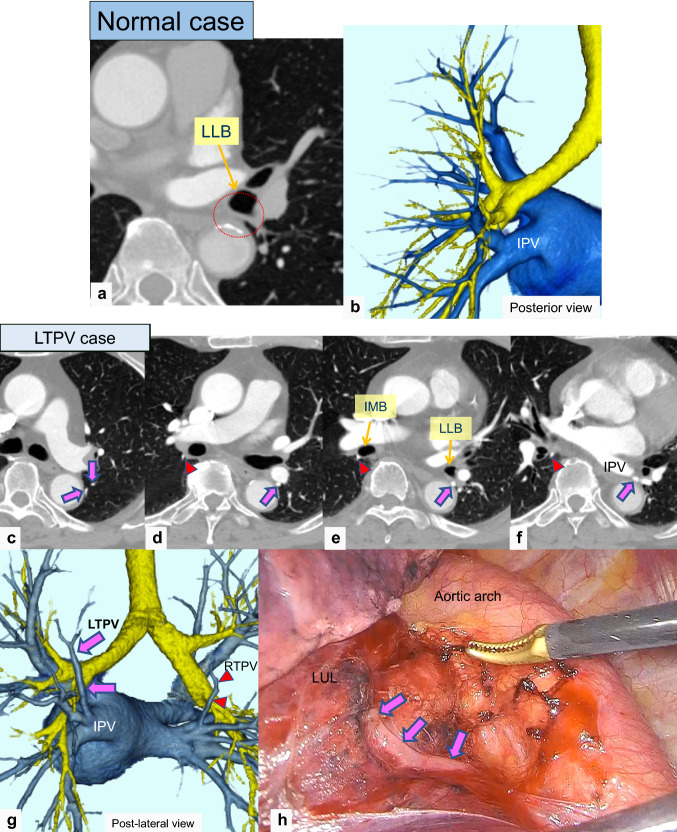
Thin-section and 3D-CTA images of a normal case and a case of left top pulmonary vein (LTPV). Thin-section CT (a) and 3D-CTA (b) images showing the normal pattern of the pulmonary vein. Thin-section CT (c–f) and 3D-CTA (g) images showing the LTPV (pink arrow) with the RTPV (red arrowhead). The intraoperative image (h) of a 70’s-year-old man with lung cancer of the left upper lobe showing the LTPV
